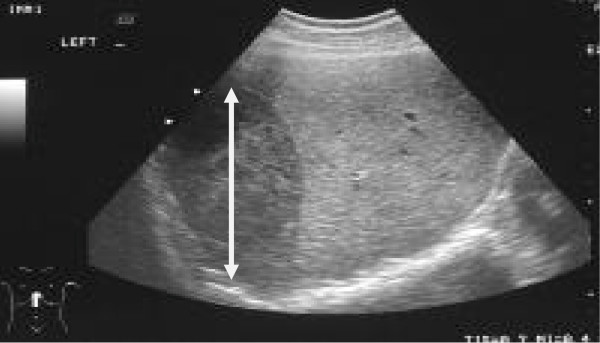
Liver ultrasound shows large haematoma (white arrow spanning the length of the hyperechoic area representing fresh blood).
